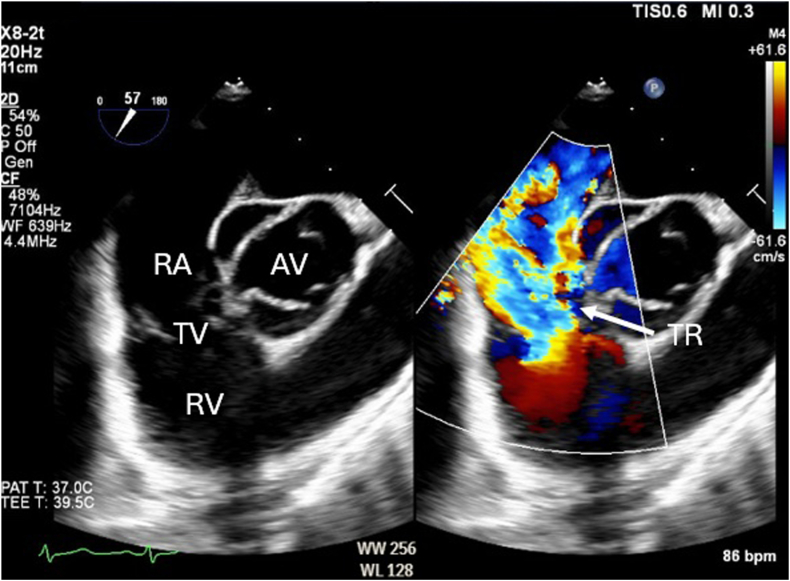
Two-dimensional TEE, midesophageal right ventricular inflow-outflow systolic view without (*left*) and with (*right*) color-flow Doppler, demonstrates severe TR and a possible interatrial shunt.
